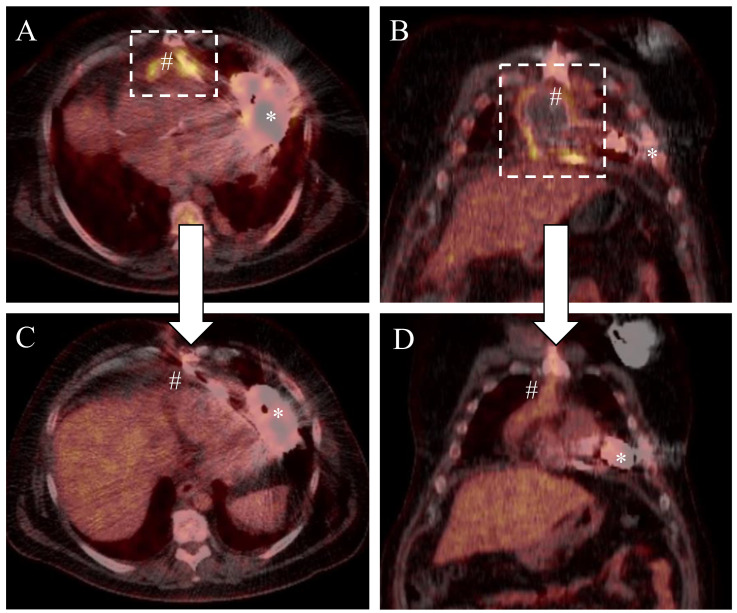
PET-CT scan before (A,B) and after (C,D) complex wound treatment. # Outflow graft of the LVAD-System; * Pump housing of the LVAD (HeartMate 3, Abbott, USA). The dashed rectangle shows contrast enhancement involving the outflow graft as a correlate for device infection.
